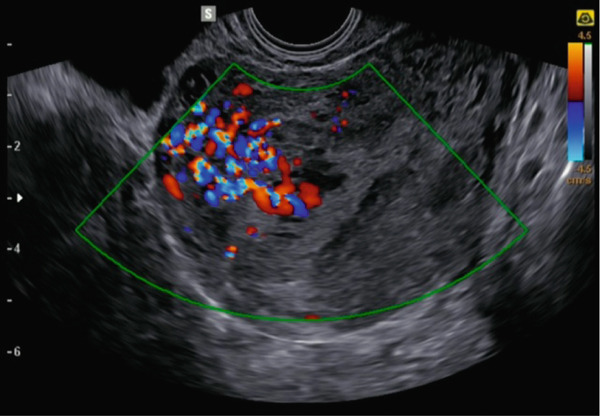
Transvaginal ultrasound revealing a heterogeneous endometrial mass with irregular borders and associated marked fundal myometrial hypervascularity on color Doppler.
